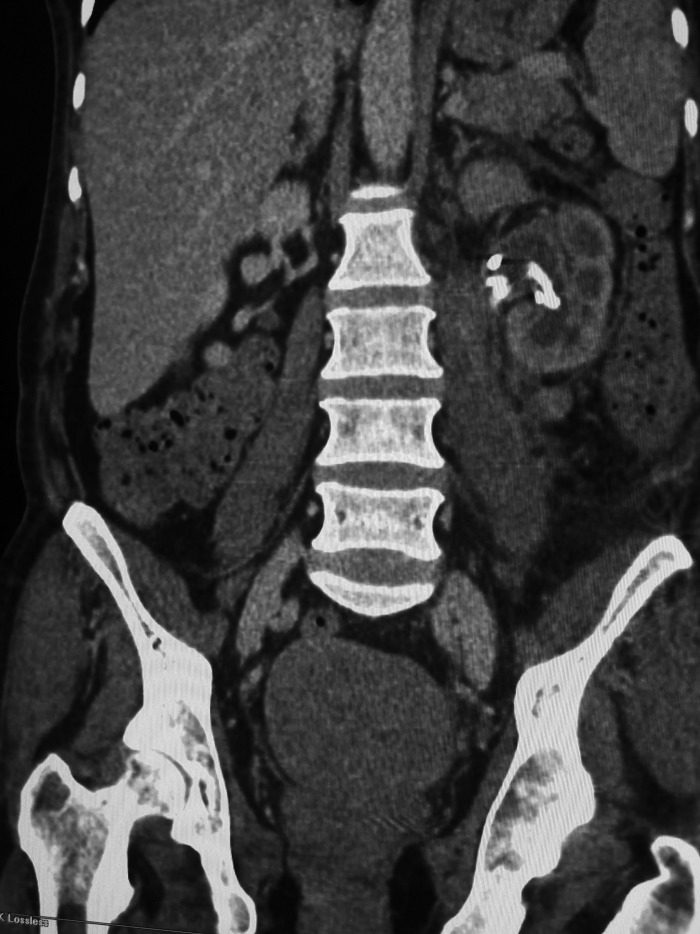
CT of the abdomen 4 days after surgical debridement, showing significant reduction of the collection with a Double-J stent in the left renal pelvis.
